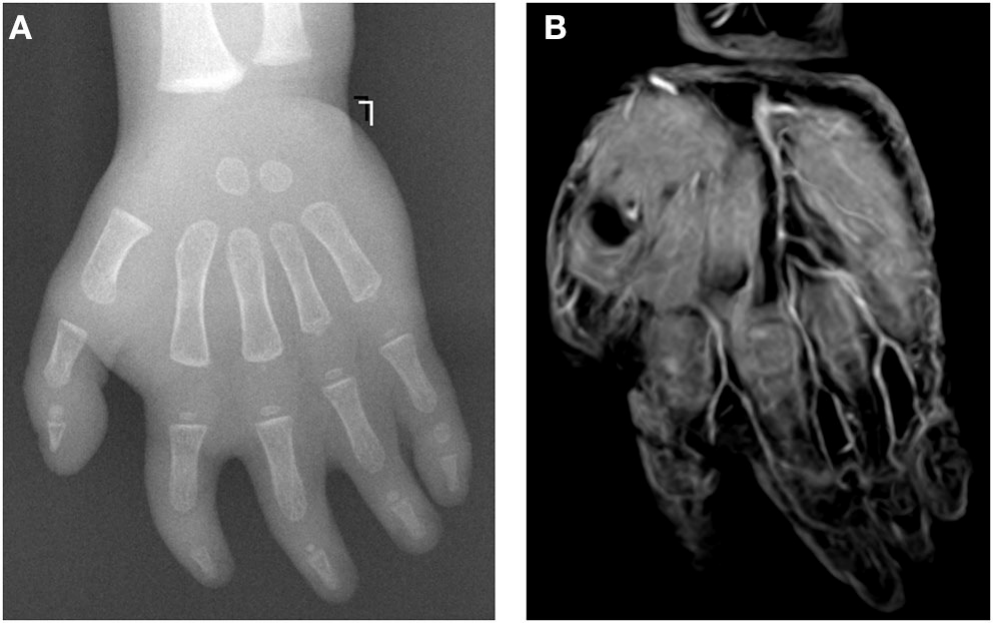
A 15-month-old female patient with complicated syndactyly of the left 2nd-3rd-4th-5th digits. (A) The preoperative radiograph showed the fused extent of soft tissues of the 2nd-3rd-4th-5th webspaces and only two phalanges of the 2nd-3rd-4th digits. (B) A contrast-enhanced three-dimensional water selective cartilage scan indicated that the bifurcation positions of three common palmar digital arteries of the hand were normal.
